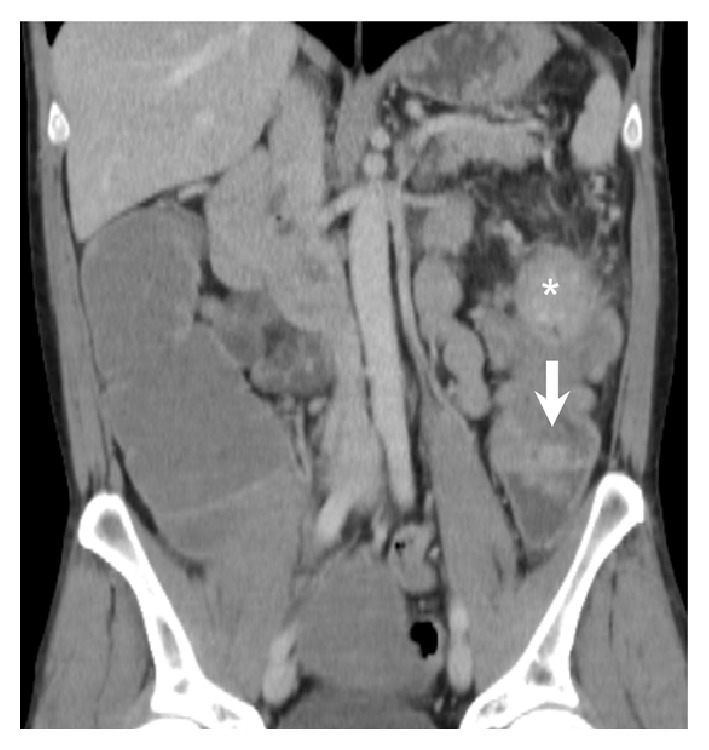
CT scan of abdomen (coronal section) showing intussusception of a polypoid lead point (*∗*) into the distal descending colon (arrow indicates direction of intussusception).
